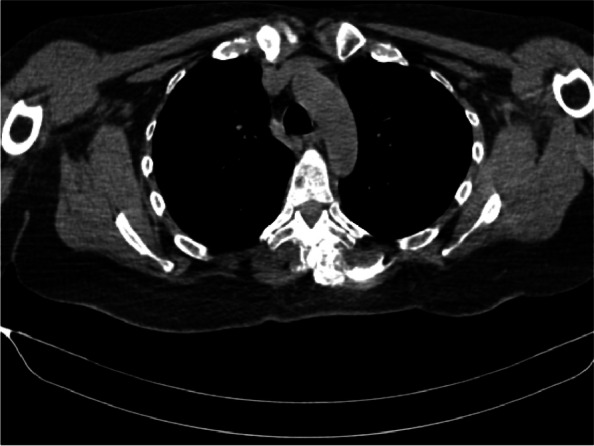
Axial CT scan showing accidental crossing of LA-dye mixture to the contralateral side at T4 level
